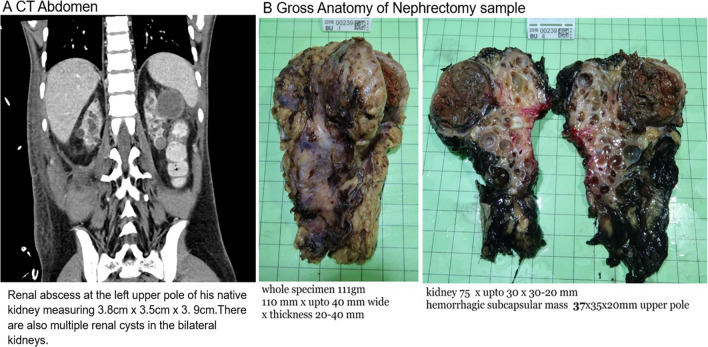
A) Urgent contrast-enhanced CT scan of the abdomen showing kidney abscess over the upper pole of the left native kidney in the background of ACKD; and B) Goss examination of the nephrectomized left native kidney revealed multiple kidney cysts with a well encapsulated subcapsular haemorrhagic roundish mass, subsequently confirmed to be ACKD-associated renal cell carcinoma
